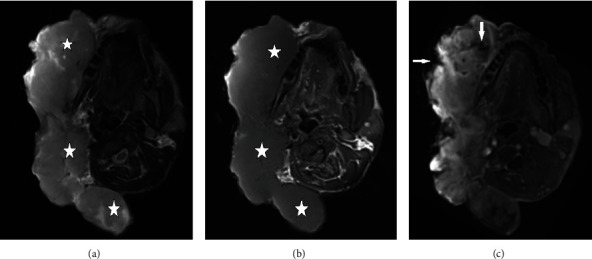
Axial MRI of the head showing a large lobulated mass centered at the right preauricular region. (a) The lesion appearing hypointense on T1-weighted imaging, (b) moderately hyperintense on T2-weighted imaging, and (c) showing marked homogeneous contrast enhancement with peripheral and intratumoral zones of necrosis (white arrows) on T1-weighted imaging after gadolinium administration. The masseter muscle and perioral muscles are not separately visible due to deep muscular invasion. There is also deep invasion of the tumour behind the right mandibular ramus in the masticator space.
